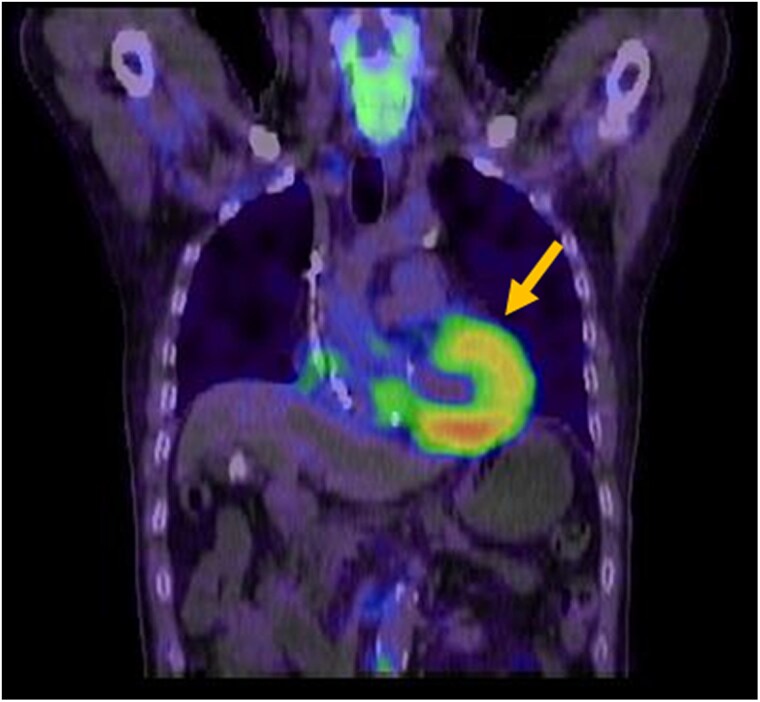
99mTc-pyrophosphate scintigraphy findings: grade 3 uptake is observed (arrow).
